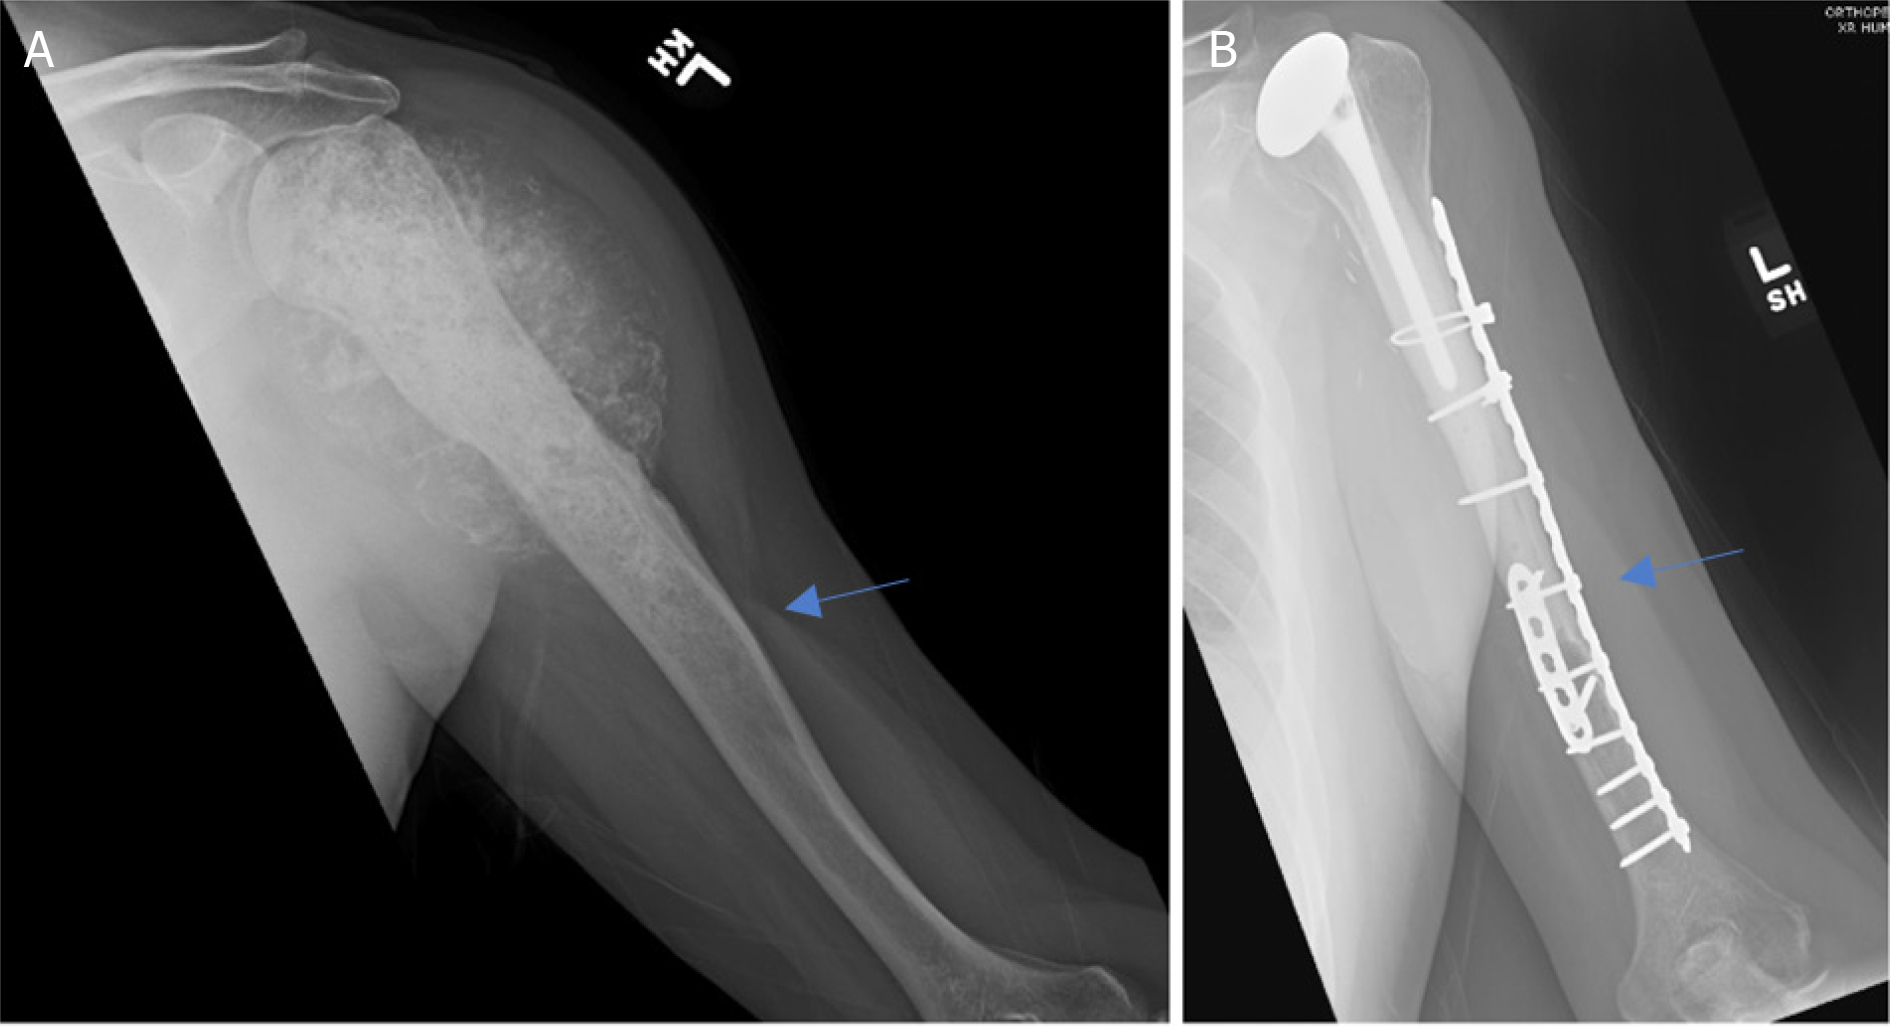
Anteroposterior radiographs of a 57-year-old male with: chondrosarcoma of the proximal humerus preoperatively (A); three years after wide excision and proximal humeral APC reconstruction (B). Blue arrow notes the junction between the allograft and native bone.
